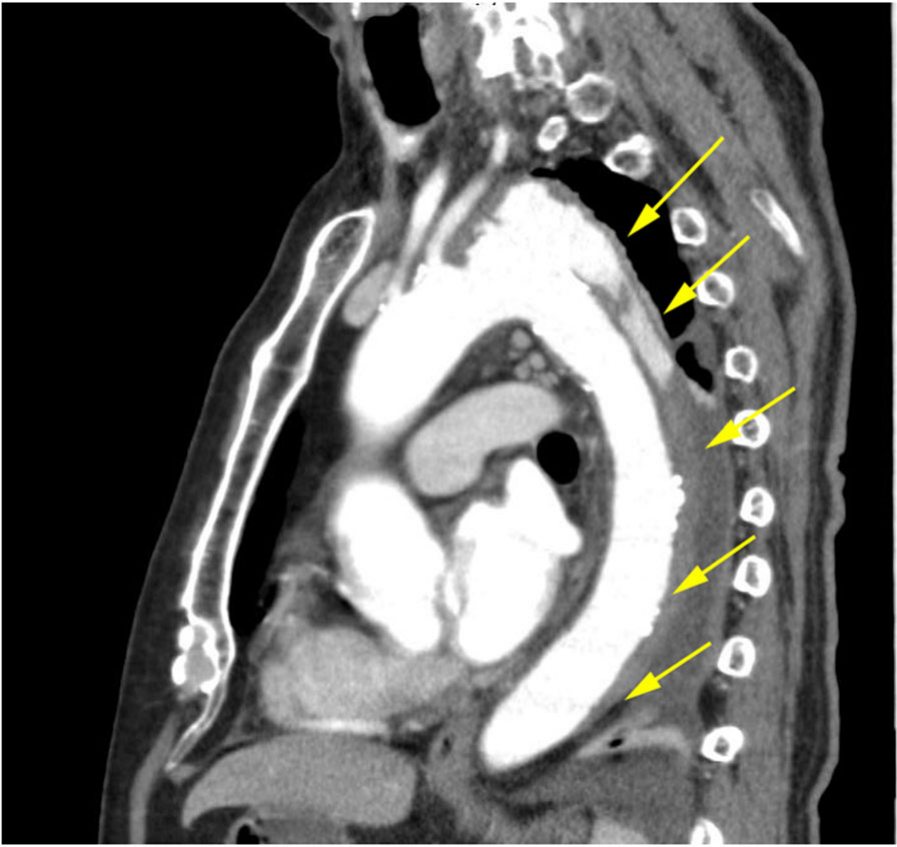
Sagittal contrast-enhanced CT chest-abdomen-pelvis image demonstrates aorta wall thickening with pseudoaneurysm of the distal aortic arch and suggestion of Stanford type B dissection involving the descending aorta, with abnormal fluid extending to the level of the diaphragm
